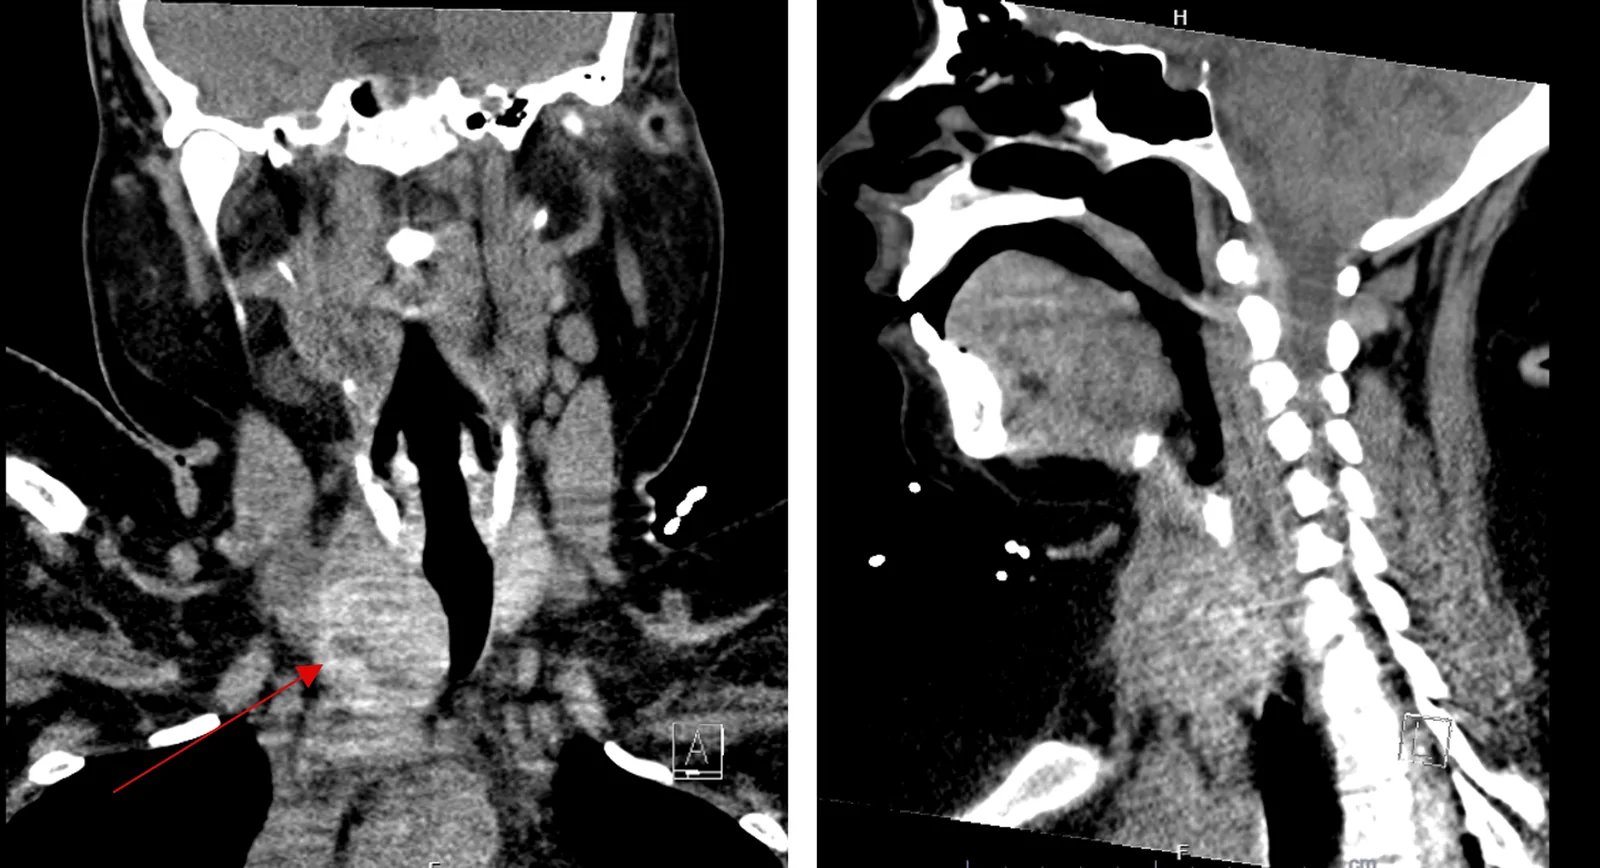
A multiphase CT scan of the neck and parathyroid (coronal, left; sagittal, right) demonstrating an enlarged, irregular right lobe of the thyroid
